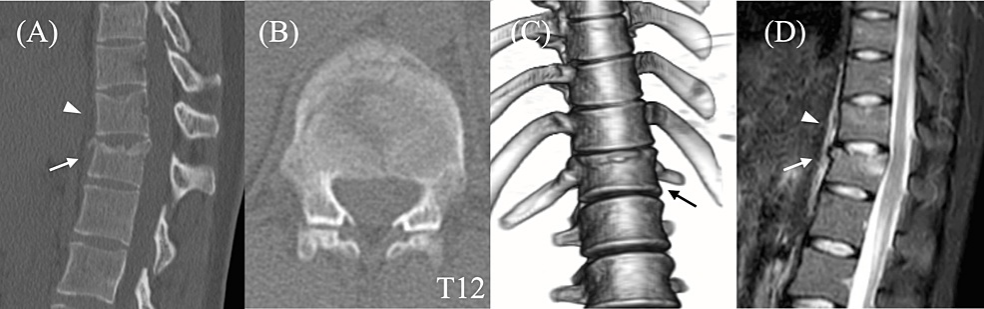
Preoperative images of the thoracolumbar spine. (A) Sagittal computed tomography; (B) axial computed tomography; (C) three-dimensional reconstruction image; and (D) sagittal short TI inversion recovery sequence in magnetic resonance imaging. T11 compression fracture (arrowhead) and T12 burst fracture (arrow).
